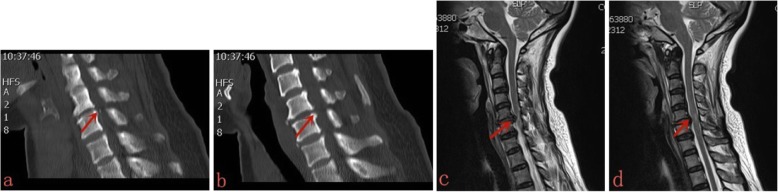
Preoperative CT images indicating that the cervical 5/6 intervertebral disc-osteophyte complex was formed(a, b); Preoperative MRI images indicating that the spinal cord was clearly compressed by disc-osteophyte complex (c), and the abnormal signal was showed (d)
